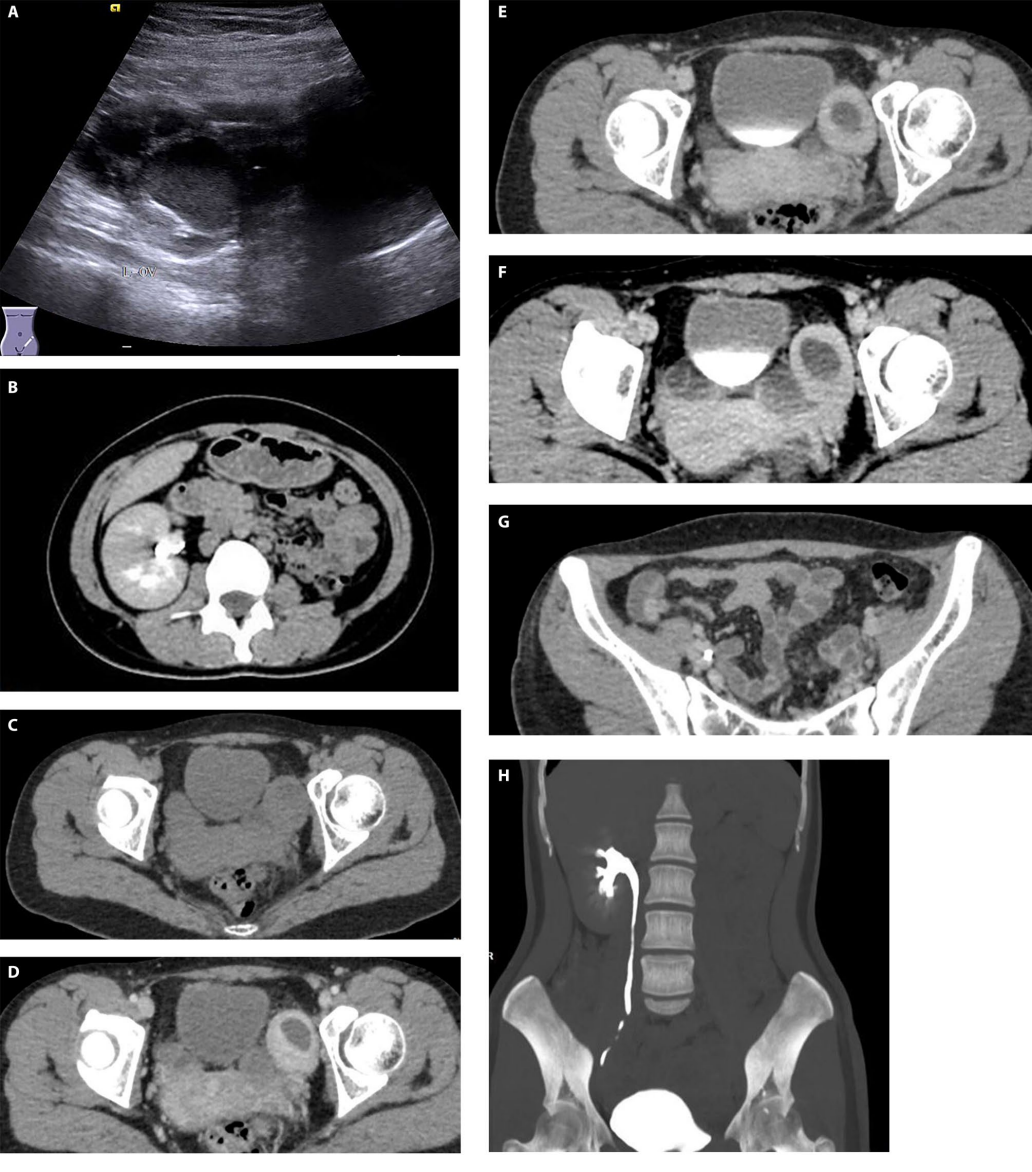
(A):Ultrasound of the left side of the pelvis showed a substantial inhomogeneous echogenic structure with clear boundary, and an irregular shape. (B,H):Computed tomography (CT) showed no indication of kidney in the left renal area. CT showed a hypodense mass of 1.6 × 2.2 cm in the left ectopic kidney. (C) :Unenhanced images. (D): Renal cortical phase. (E) :Renal corticomedullary phase. (F):Renal excretory phase. (G): the ureter can be seen as dilated and hydrous in the left ectopic kidney
